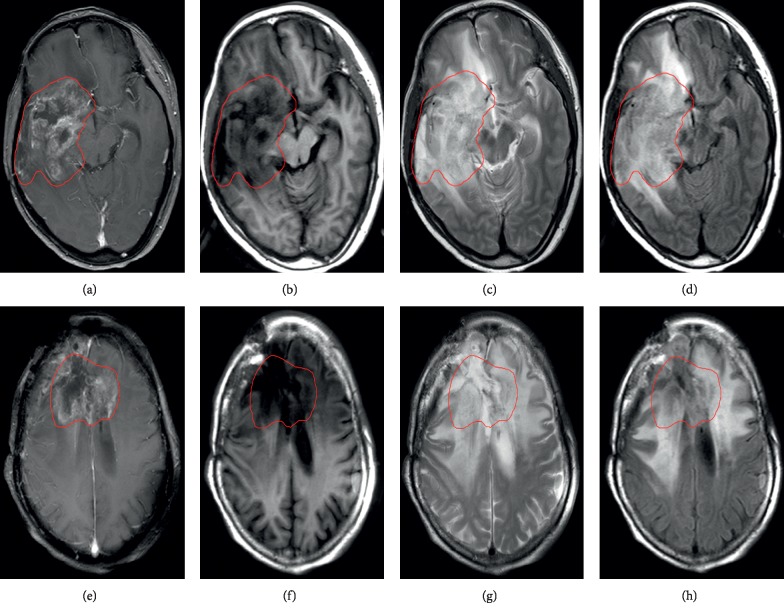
MRI diagnostic images of two patients with glioma. (a–d) Recurrent image of a 52-year-old patient with astrocytoma 1 year after radiotherapy. (e–h) Necrotic image of a 54-year-old patient with oligodendroglioma 6 months after radiotherapy. (a), (b), (c), and (d) and (e), (f), (g), and (h), respectively, show T1C, T1, T2, and FLAIR images. The inside of the red line shows the edge of the lesion.
